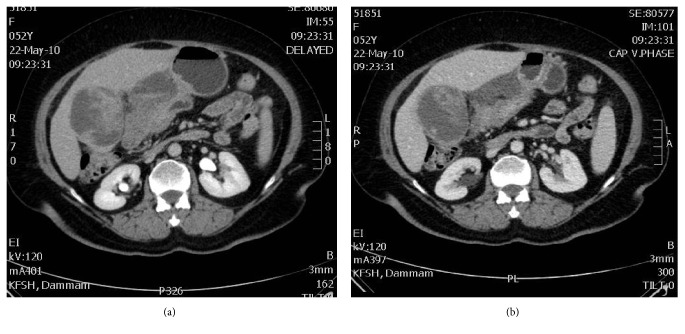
(a) and (b) Abdominal CT scan demonstrating a large exophytic mass within the gallbladder.
